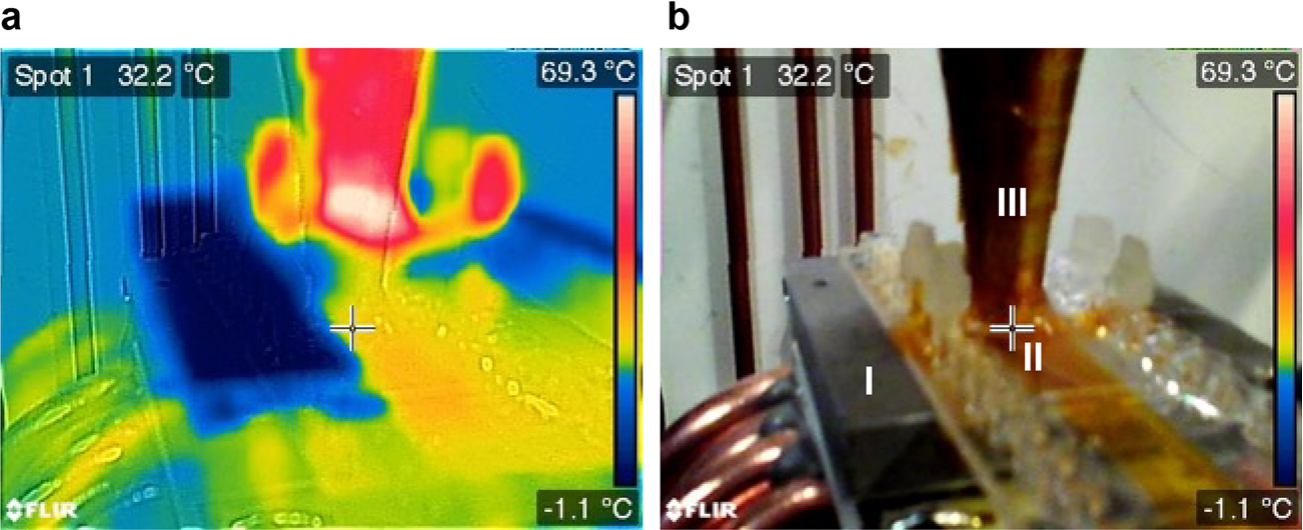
Thermal infrared image (a) and real visible-light image (b) of the system after 1 min of ultrasonic actuation. The system includes (*I*): The Peltier-cooled aluminum heat sink acting as the chip holder, (*II*): The chip, and (*III*): The exponential horn of the Langevin-type ultrasonic transducer. The orange part of the chip in (b) is a tape used for the thermal imaging camera (cf. Fig. 1a)
